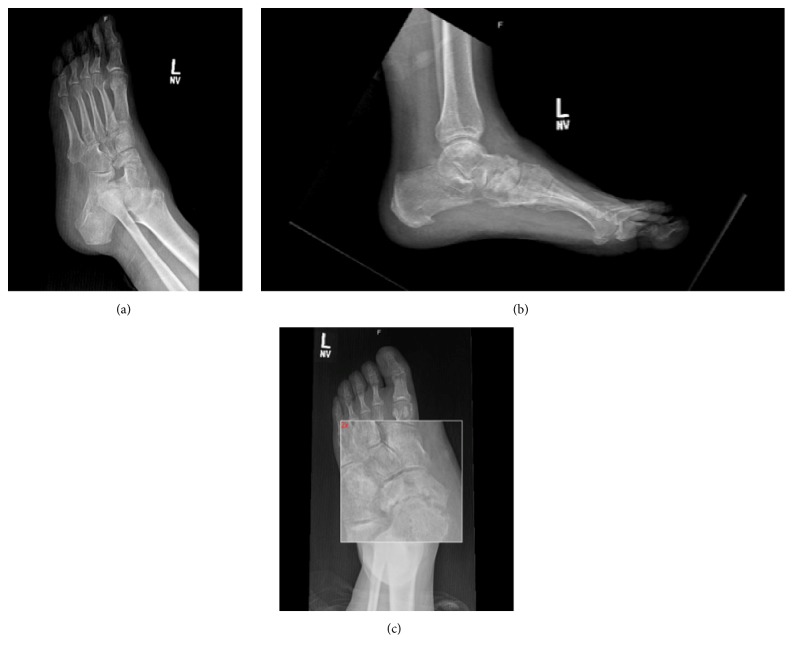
Final radiographs demonstrating development of talonavicular arthritis.
